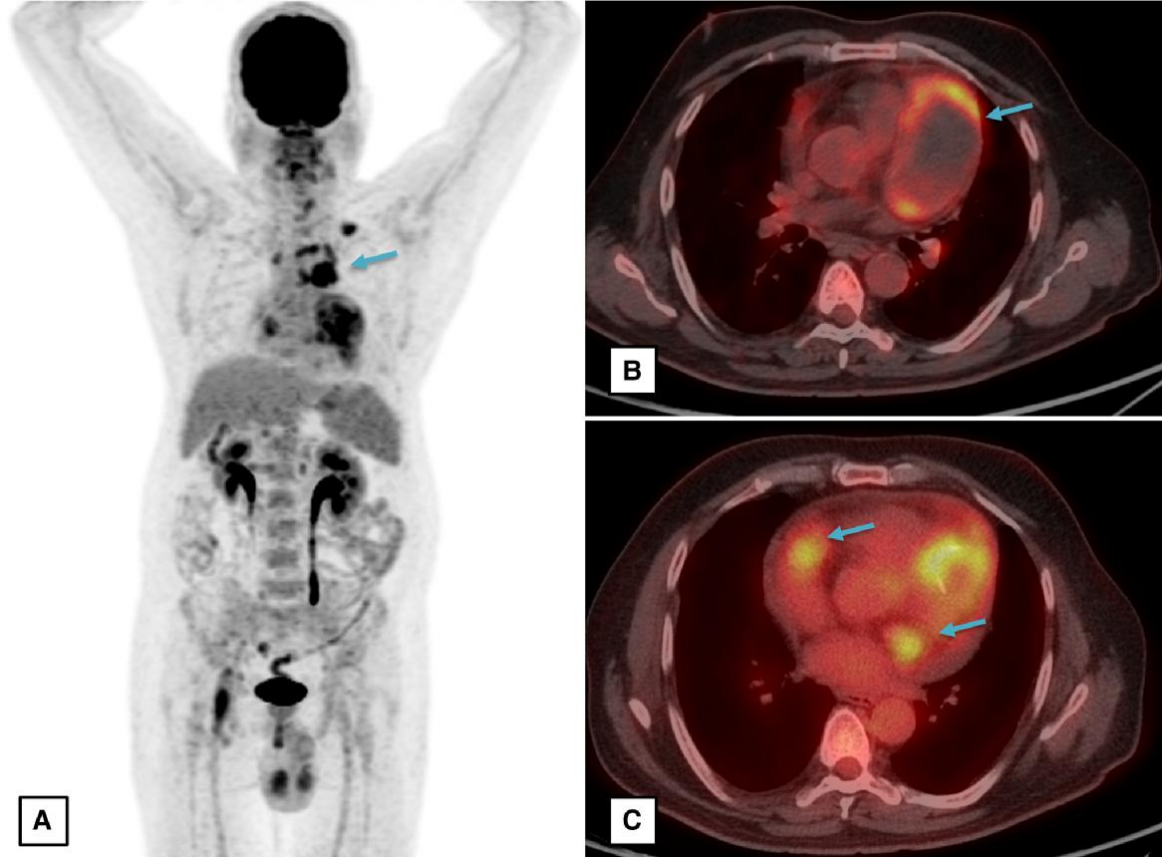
Fluorodeoxyglucose-positron emission tomography (FDG-PET) images. (*A*) Whole body coronal section, (*B* and *C*) transverse section at aortic sinus and cardiac level, respectively. Images showing significant FDG uptake (star *) in the perivascular soft tissue masses including the wall of left anterior descending (LAD) artery pseudoaneurysm.
